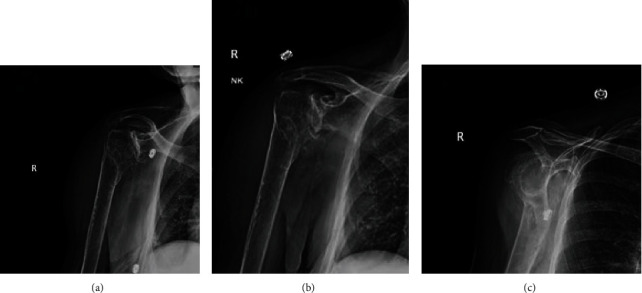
(a–c) Plain radiographs of the right shoulder showing a displaced proximal humerus fracture involving the surgical neck and greater tuberosity.
